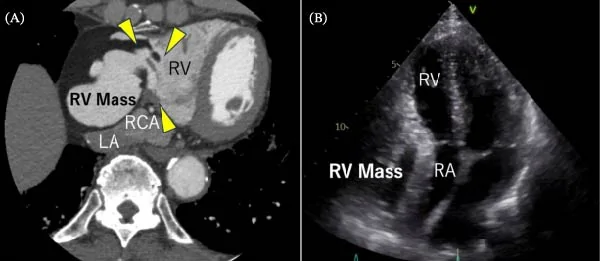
Preoperative imaging. CT revealing a 47 mm × 76 mm mass with multiple slit‐like communications connecting the RV and mass (arrowhead). The predominantly adipose composition of the mass wall suggests a lipoma (A). Transthoracic echocardiography demonstrating that the mass in the RV compressed and deformed the RA (B), with evidence of to‐and‐fro blood flow between the RV and mass. CT, computed tomography; RA, right atrium; RV, right ventricle.
